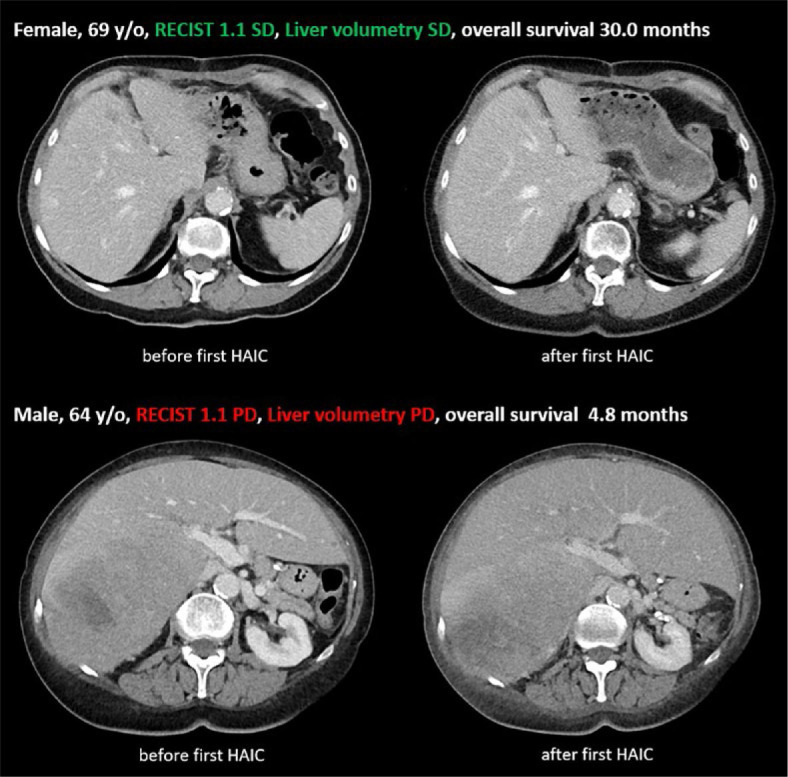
Image examples of CT examinations in portal venous phase before and after first hepatic artery infusion chemotherapy (HAIC) for two patients with evaluations according to RECIST 1.1 and liver volumetry (LV) as stable disease (SD) and progressive disease (PD). Liver metastases are marked with arrows.
